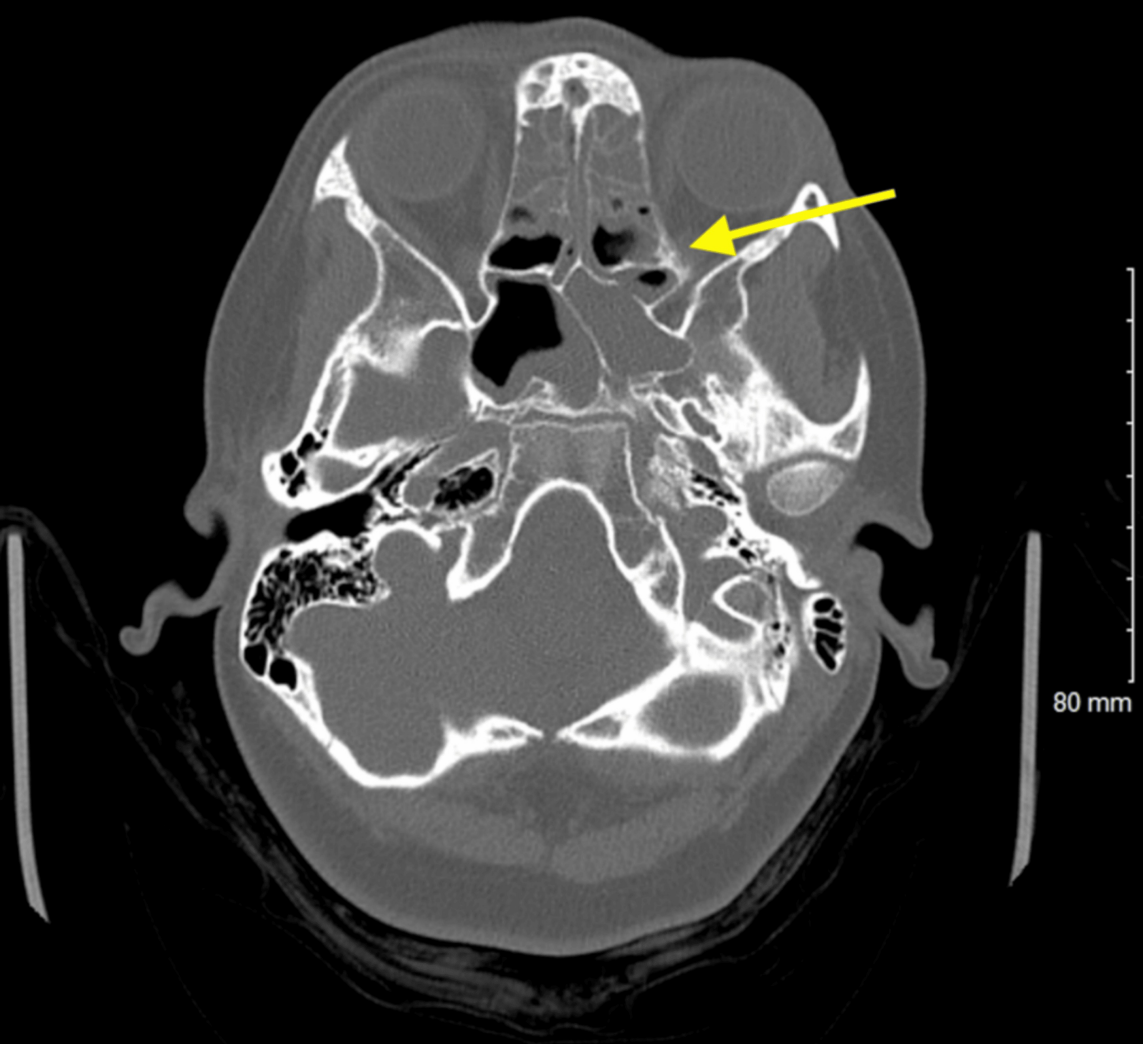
Unenhanced axial computed tomography image of the brain demonstrates extensive inflammatory changes in the sinuses bilaterally.
